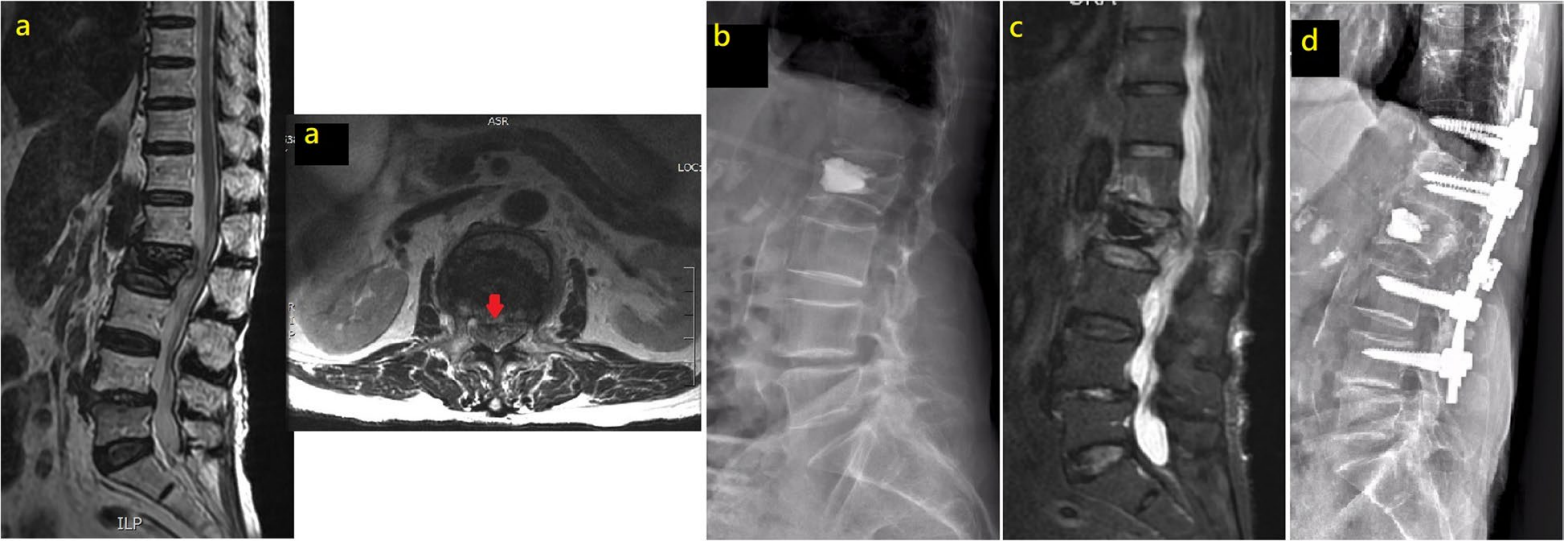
MRI of L2 compression fracture with posterior vertebral rim disruption (red arrow) (a). L2 PKP was performed, left L2 radiculopathy developed 2 weeks after surgery (b). MRI showing further protrusion of the posterior vertebral fragment posteriorly (c). Surgical decompression and T12-L4 instrumentation were performed (d)
